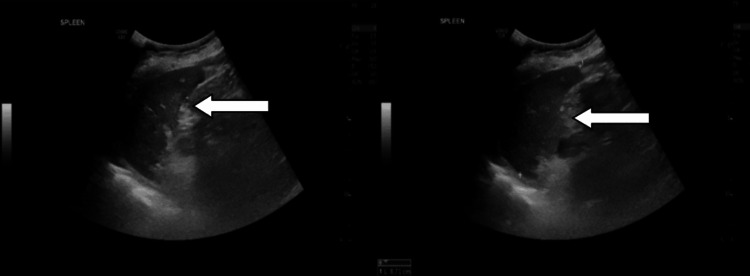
Ultrasound image obtained before colonoscopy showing the spleen (arrows) with normal appearance.
